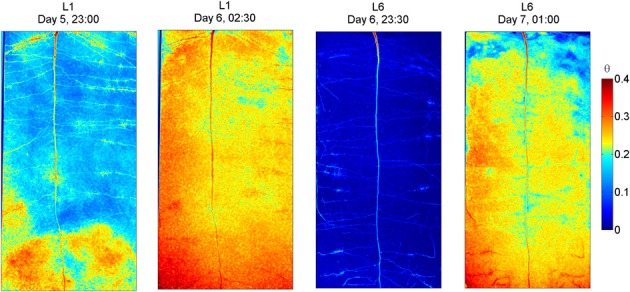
Neutron radiography of L1 and L6 before and after irrigation. L1 was irrigated every second day. Before irrigation its average water content was 0.17. After irrigation, the rhizosphere of the upper and middle part was not fully rewetted. Low and young roots were rewetted. L6 experienced a longer and more pronounced drying and was irrigated when its water content was 0.02 and the plant showed wilting symptoms. After irrigation the rhizosphere of most roots was not rewetted, except at the root tips, which appeared being covered with a blob of wet material.
